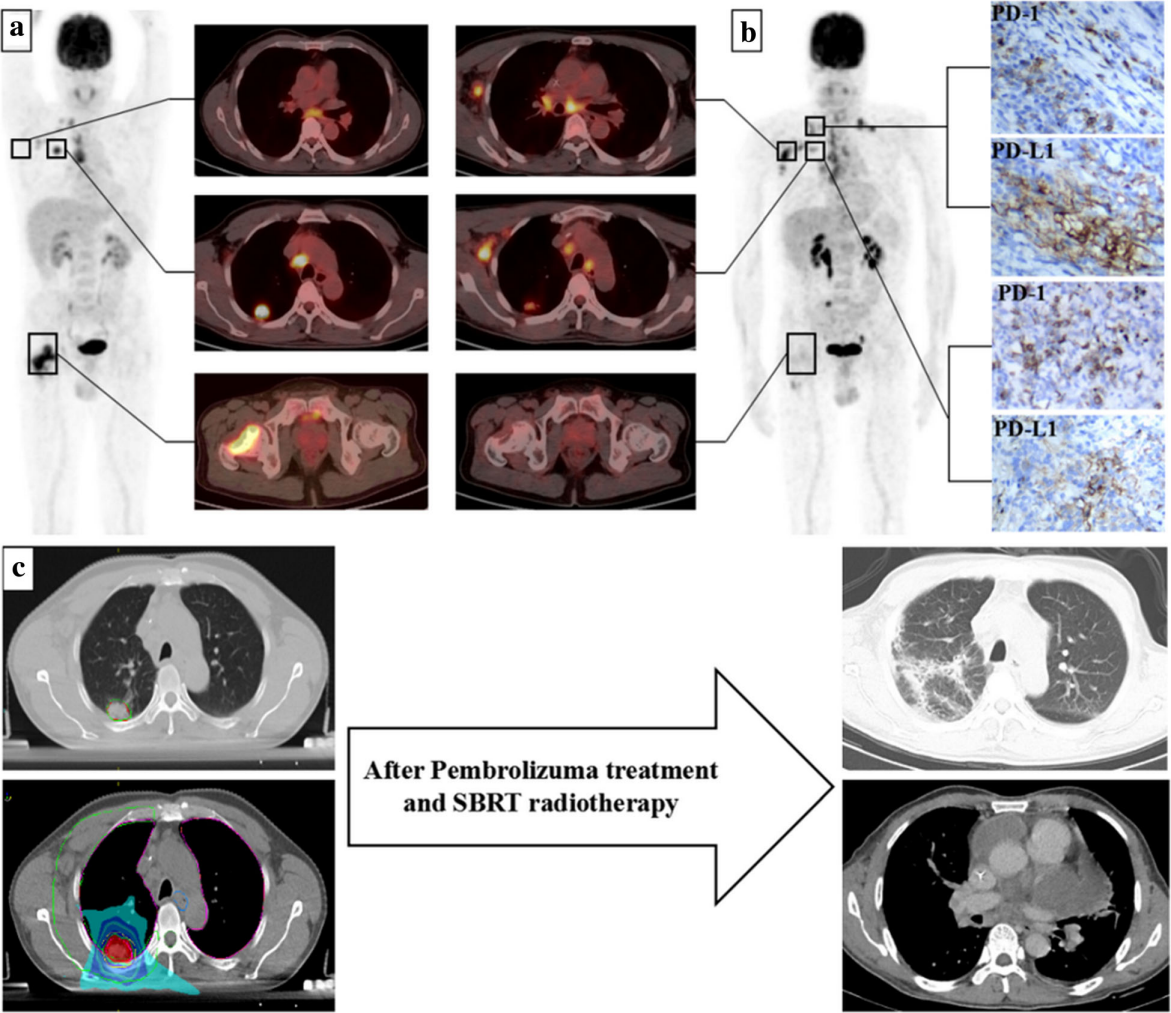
a Before treatment, tumors of the lung (posterior segment of upper lobe of right lung) and the ipsilateral femoral neck, as well as a small amount of axillary and cervical lymph nodes, were found by positron emission tomography-computed tomography. b After chemoradiotherapy, the tumors in the lung and femoral neck were controlled. However, the tumor of axillary and cervical lymph nodes progressed. Meanwhile, immunochemistry detection showed that programmed death-1 and programmed death-ligand 1 expression were more than 50% in the tumor cells and immune cells of biopsy tissues of the cervical lymph node and primary lung tumor. c After three cycles of pembrolizumab and 5 weeks of stereotactic body radiotherapy therapy, the patient presented with pericardial effusion and interstitial pneumonia. *PD-1* programmed death-1, *PD-L1* programmed death-ligand 1, *SBRT* stereotactic body radiotherapy
